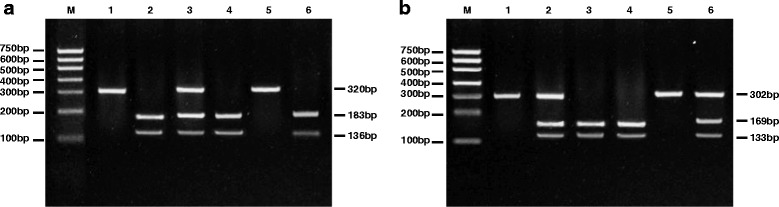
Electrophoresis map of *CD40* rs4810485 G > T and rs1883832 C > T. Note: a, *CD40* rs4810485 G > T polymorphism: 1 and 5, GG genotype; 2 and 4, TT genotype; 3 and 6, GT genotype; b, *CD40* rs1883832 C > T polymorphism: 1 and 5, TT genotype; 2 and 6, CT genotype; 3 and 4, CC genotype; *CD40*, Cluster of Differentiation 40
